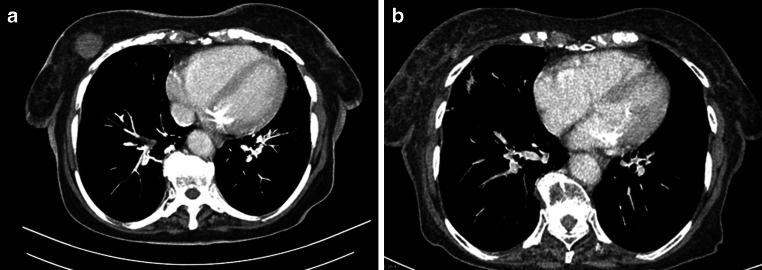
Local tumor remission on CT imaging: a before radiotherapy, b 3 months after radiotherapy. *CT* computer tomography
